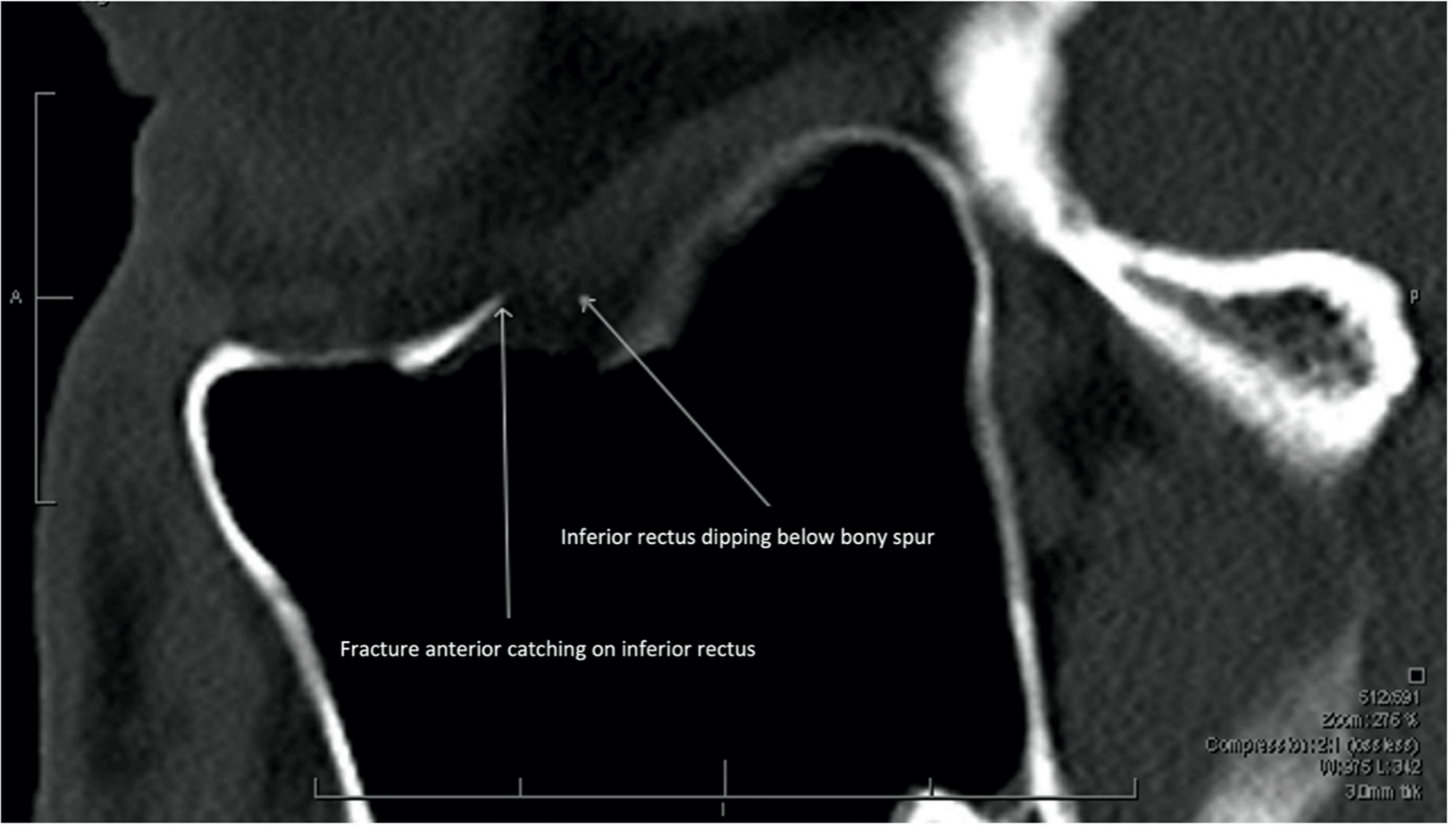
Parasagittal CT Orbits (Bony Windows), Prior to Forced Duction Testing. Note the anterior lip of the fractured bone impinging on the anteroinferior aspect of the inferior rectus, with modest orbital fat herniation.
